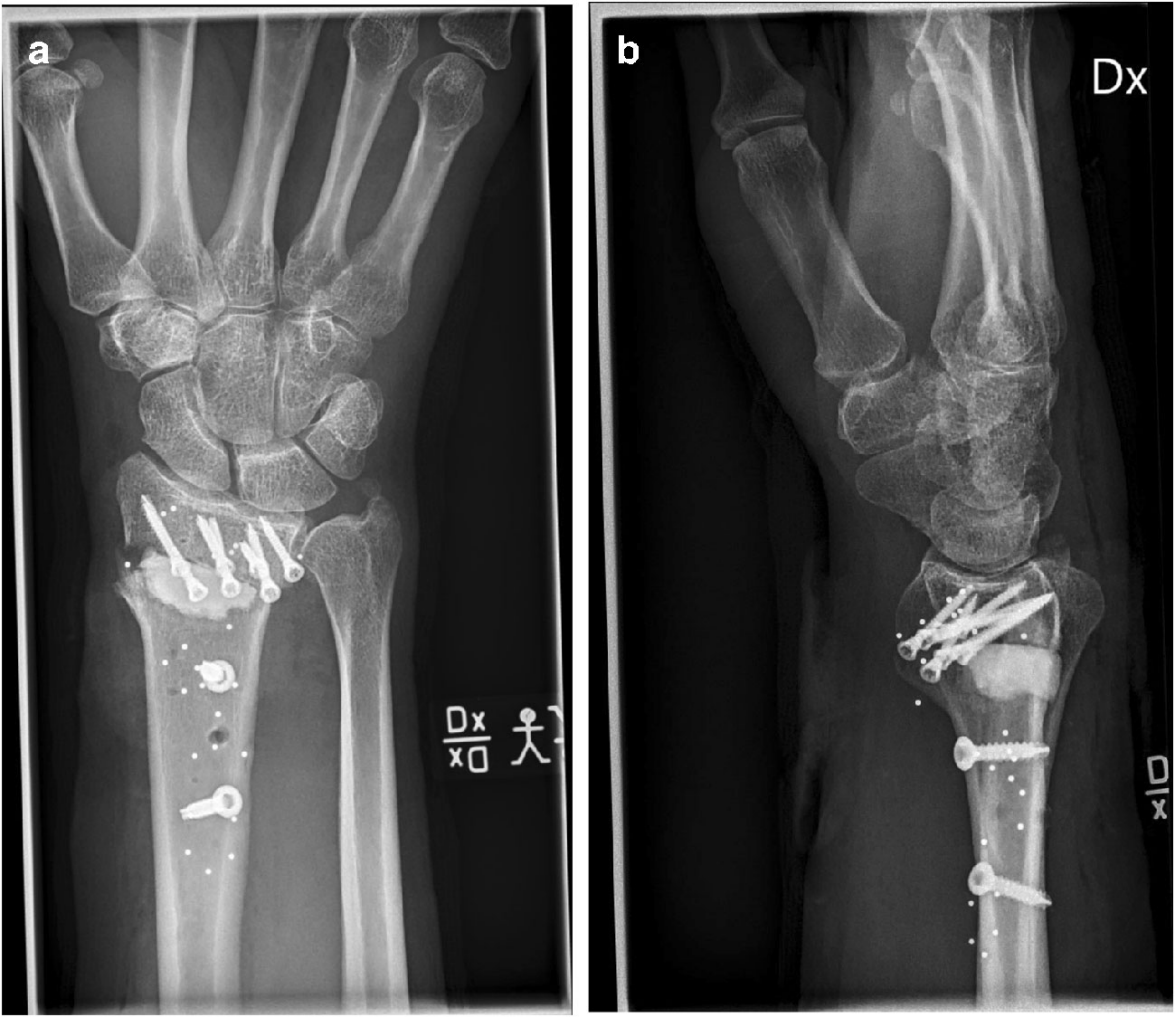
Post-operative radiographs after open osteotomy using bone substitute. a AP view. b Sagittal view
